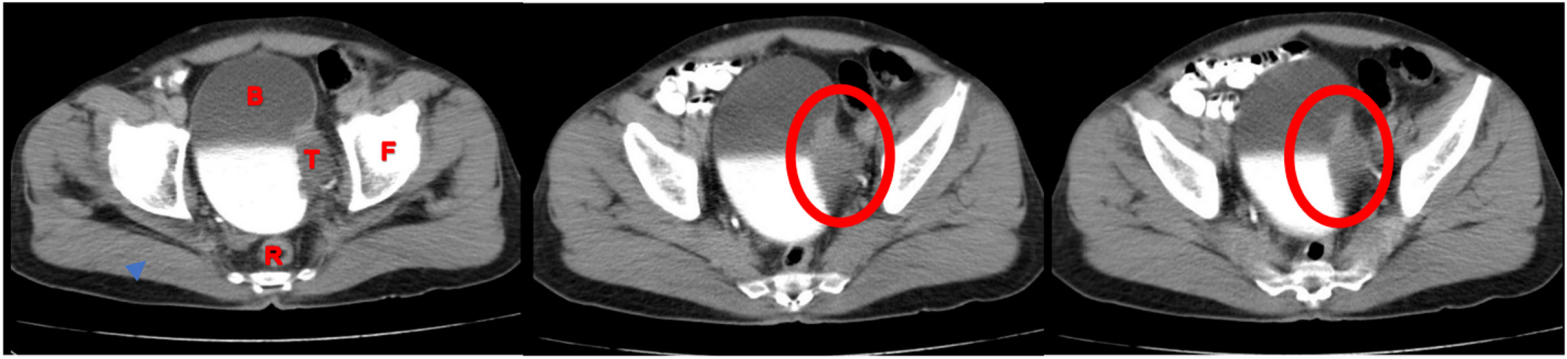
CT scan of pelvis shows an intravesical mass (red ellipse and T word indicate the tumoral mass) on the left lateral border of the bladder (B: bladder, T: tumoral mass, R: rectum, F: femur head)
